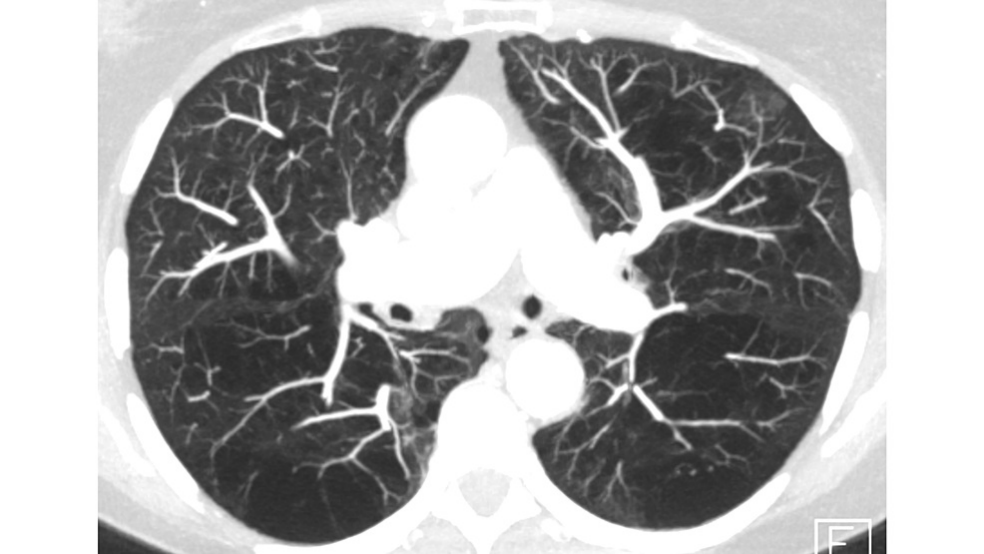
Axial CT scan (September 2021) corresponding to the baseline image before treatment (RECIST criteria: complete remission) CT, computed tomography
